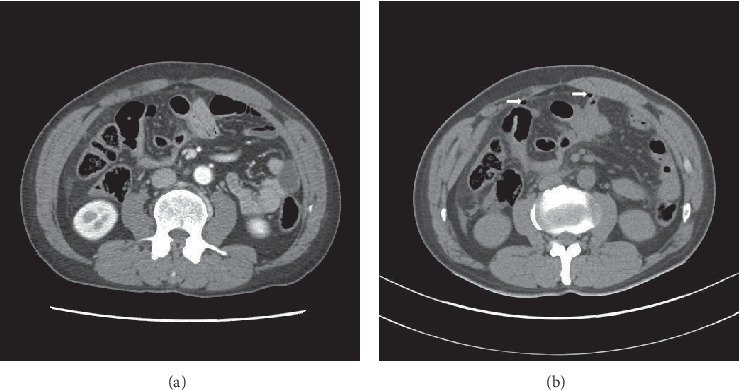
A 40-year-old man with blunt abdominal trauma due to assault. (a) Axial contrast-enhanced CT scan shows focal small bowel ileus in the LUQ. (b) Follow-up CT scan was performed after 4 hours. Axial unenhanced CT scan shows small air bubbles (white arrow) between the bowel lumen and the peritoneal cavity.
